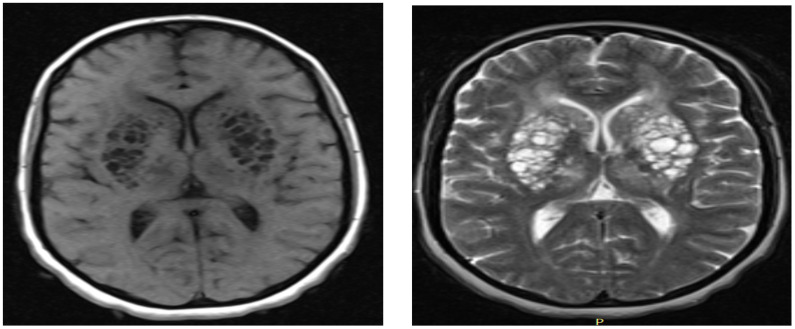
a) Axial T1 weighted MRI showing bilateral multiple hyporintense cystic lesions in basal ganglia region having mass effect on adjacent ventricles by making it like a slit; b) Axial T2 weighted MRI showing bilateral multiple hyporintense cystic lesions in basal ganglia region having mass effect on adjacent ventricles making it slit
